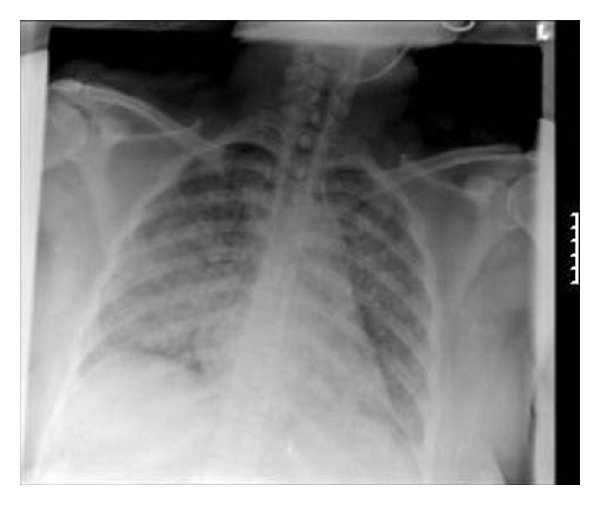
Chest radiograph on admission showing bilateral interstitial disease with alveolar filling (worse on the right side).
